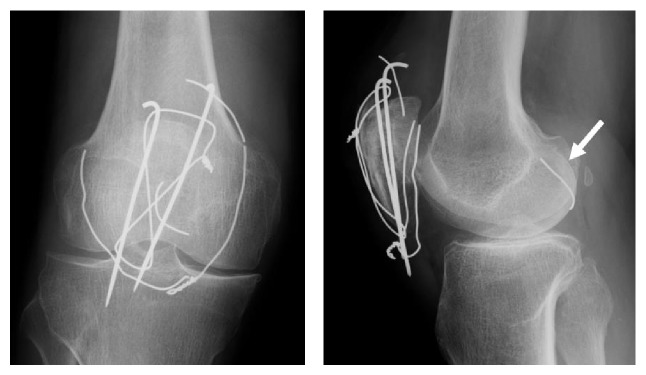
Plain radiographs of the right knee show a fragment of wire migrated into the posterior compartment of the right knee (arrow).
